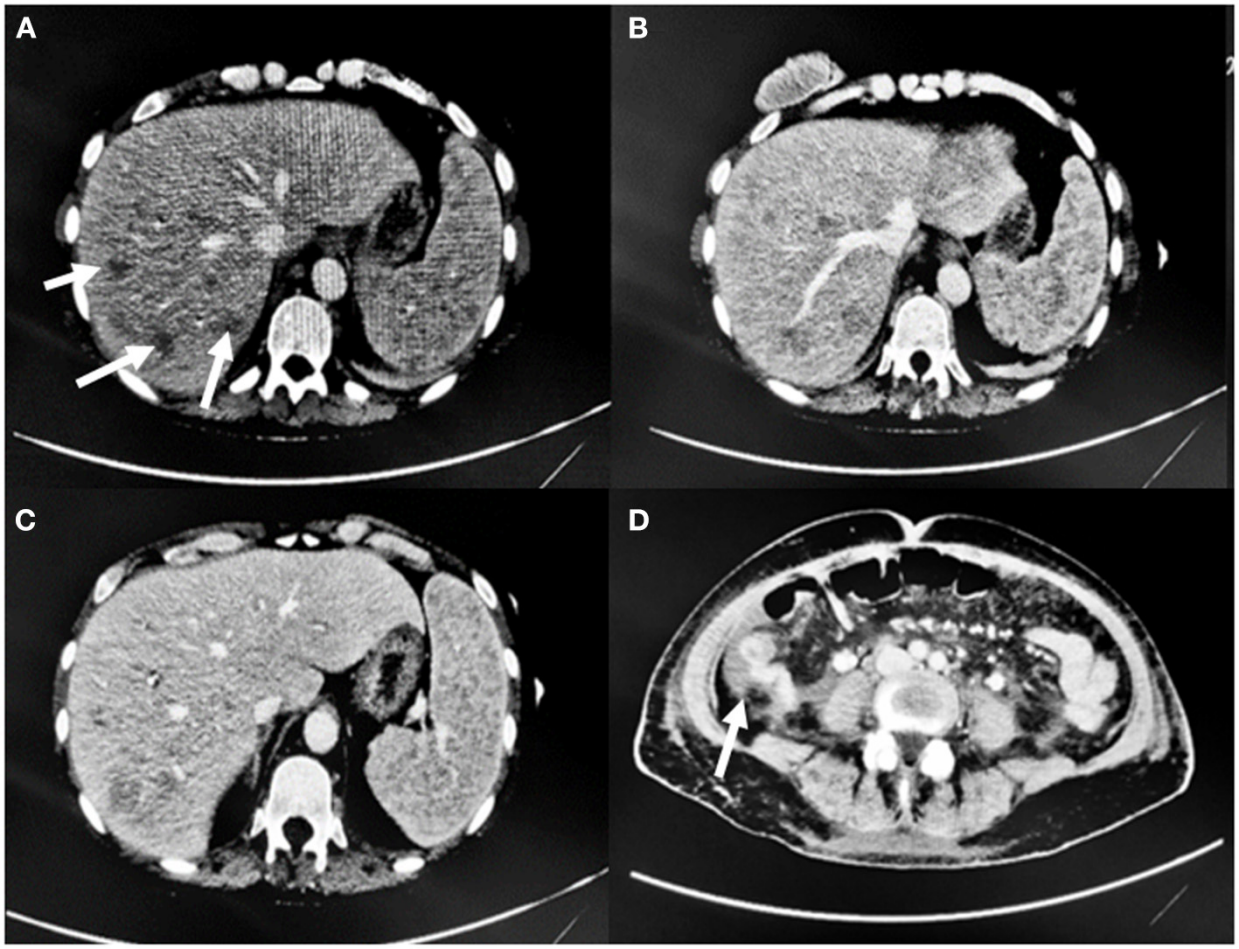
CT scan findings. (A, B) Increase in size and number of hepatic lesions (arrows), (C) Splenomegaly. (D) Fluid effusion in the right colic gutter.
